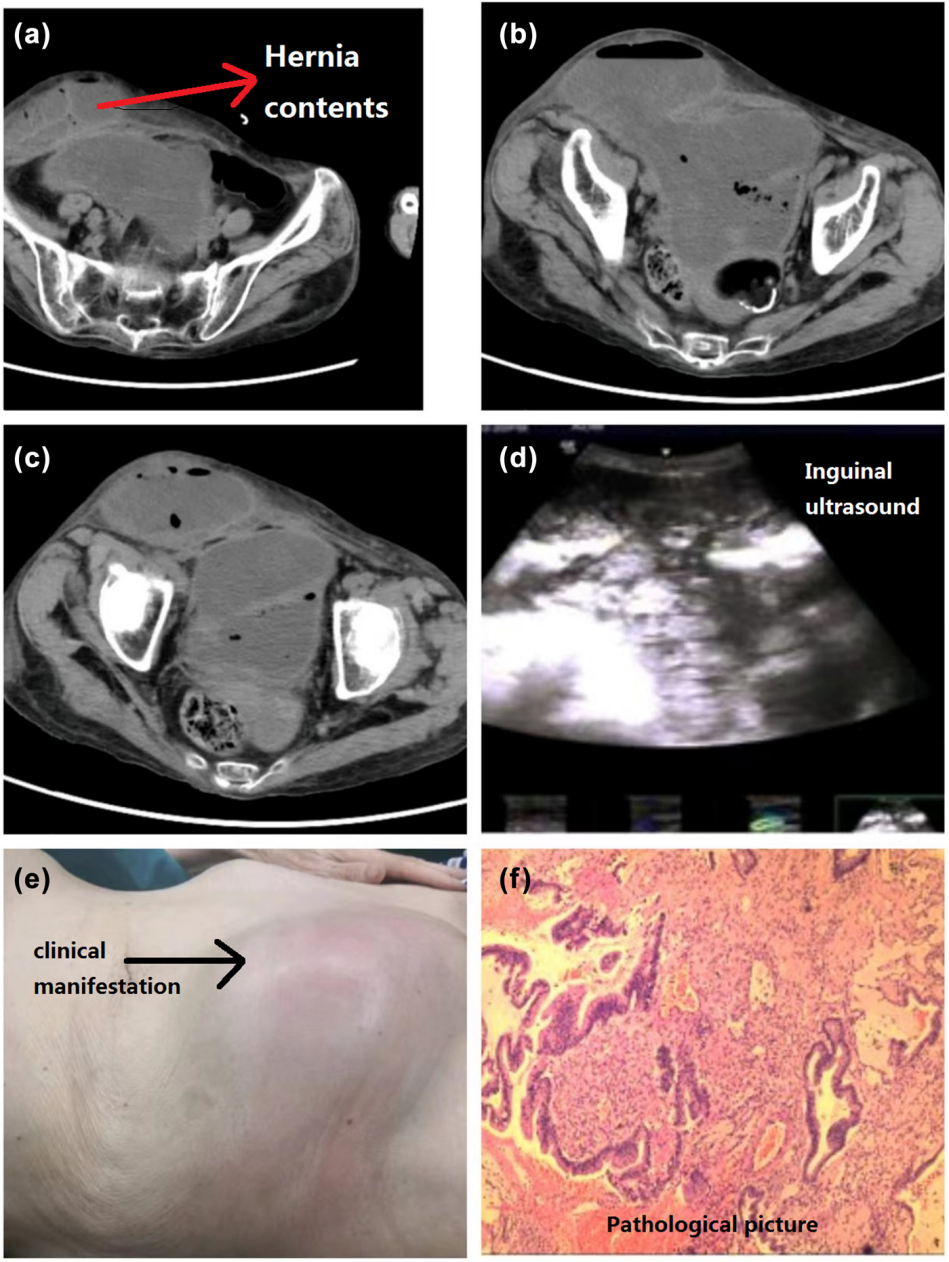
(a–c) Hernia contents; (d) inguinal ultrasound; (e) clinical manifestation; and (f) pathological picture.
